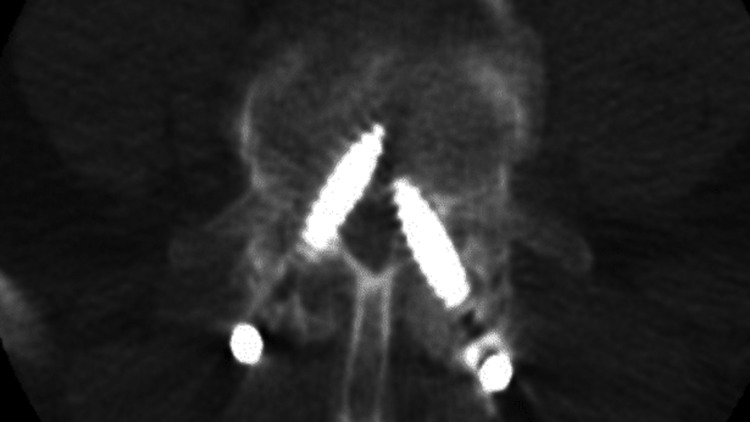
Postoperative CT scan demonstrating malposition of both L4 screws with a medial breach.
